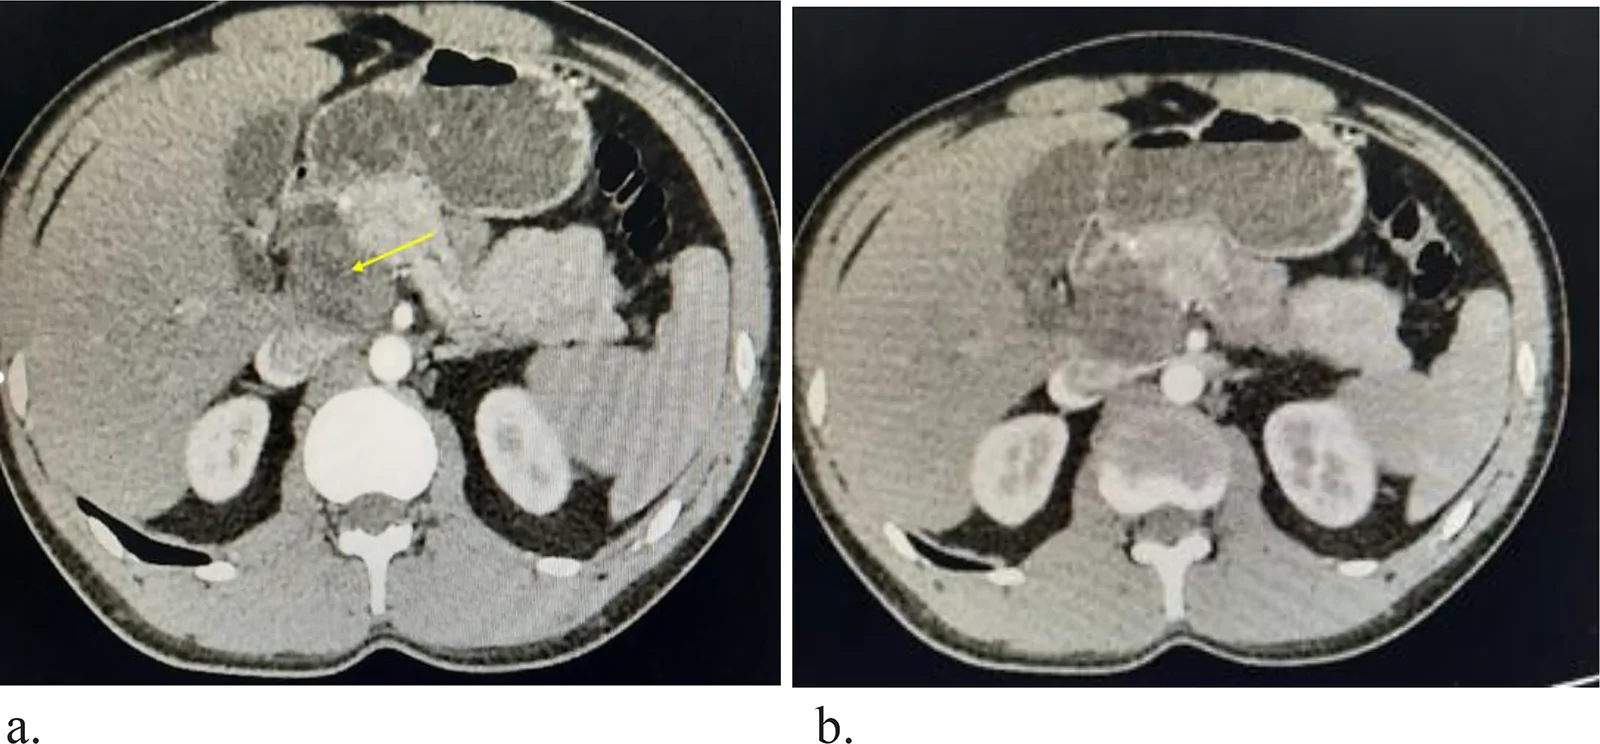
(a, b) CT scan with contrast of the abdomen.Arrow in the Fig 1a points to a heterogeneously enhancing hypodense lobulated mass in the uncinate process and the head of the pancreas
